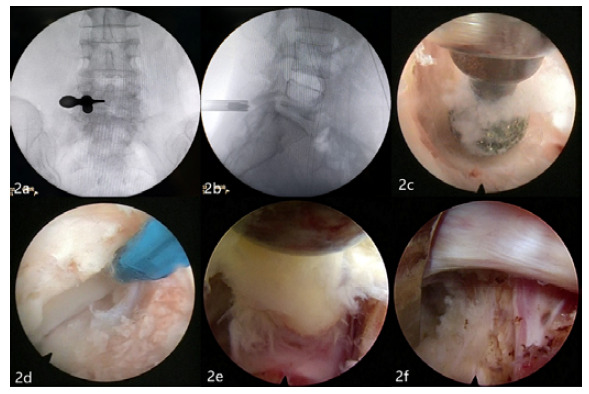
Procedures for full-endoscopic visualization via the interlaminar approach. Intraoperative anteroposterior and lateral radiographs confirmed the intervertebral gap and interlaminar space (a, b); Fenestration of interlaminar spaces on the affected side was achieved by a power drill (c); The insertion of the ligamentum flavum is exposed from the inner edge of the superior articular process (d); The outer edge of the autonomic nerve root was exposed inwardly and the prominent nucleus pulposus tissue was removed (e); Exploration of nerve root relaxation with no nucleus pulposus left (f).
